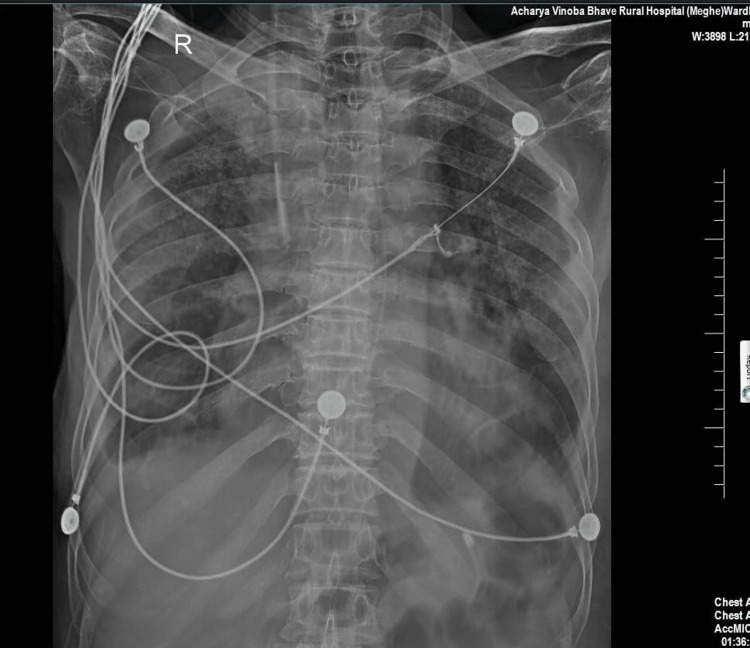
The patient's chest x-ray shows bilateral pulmonary infiltrates
